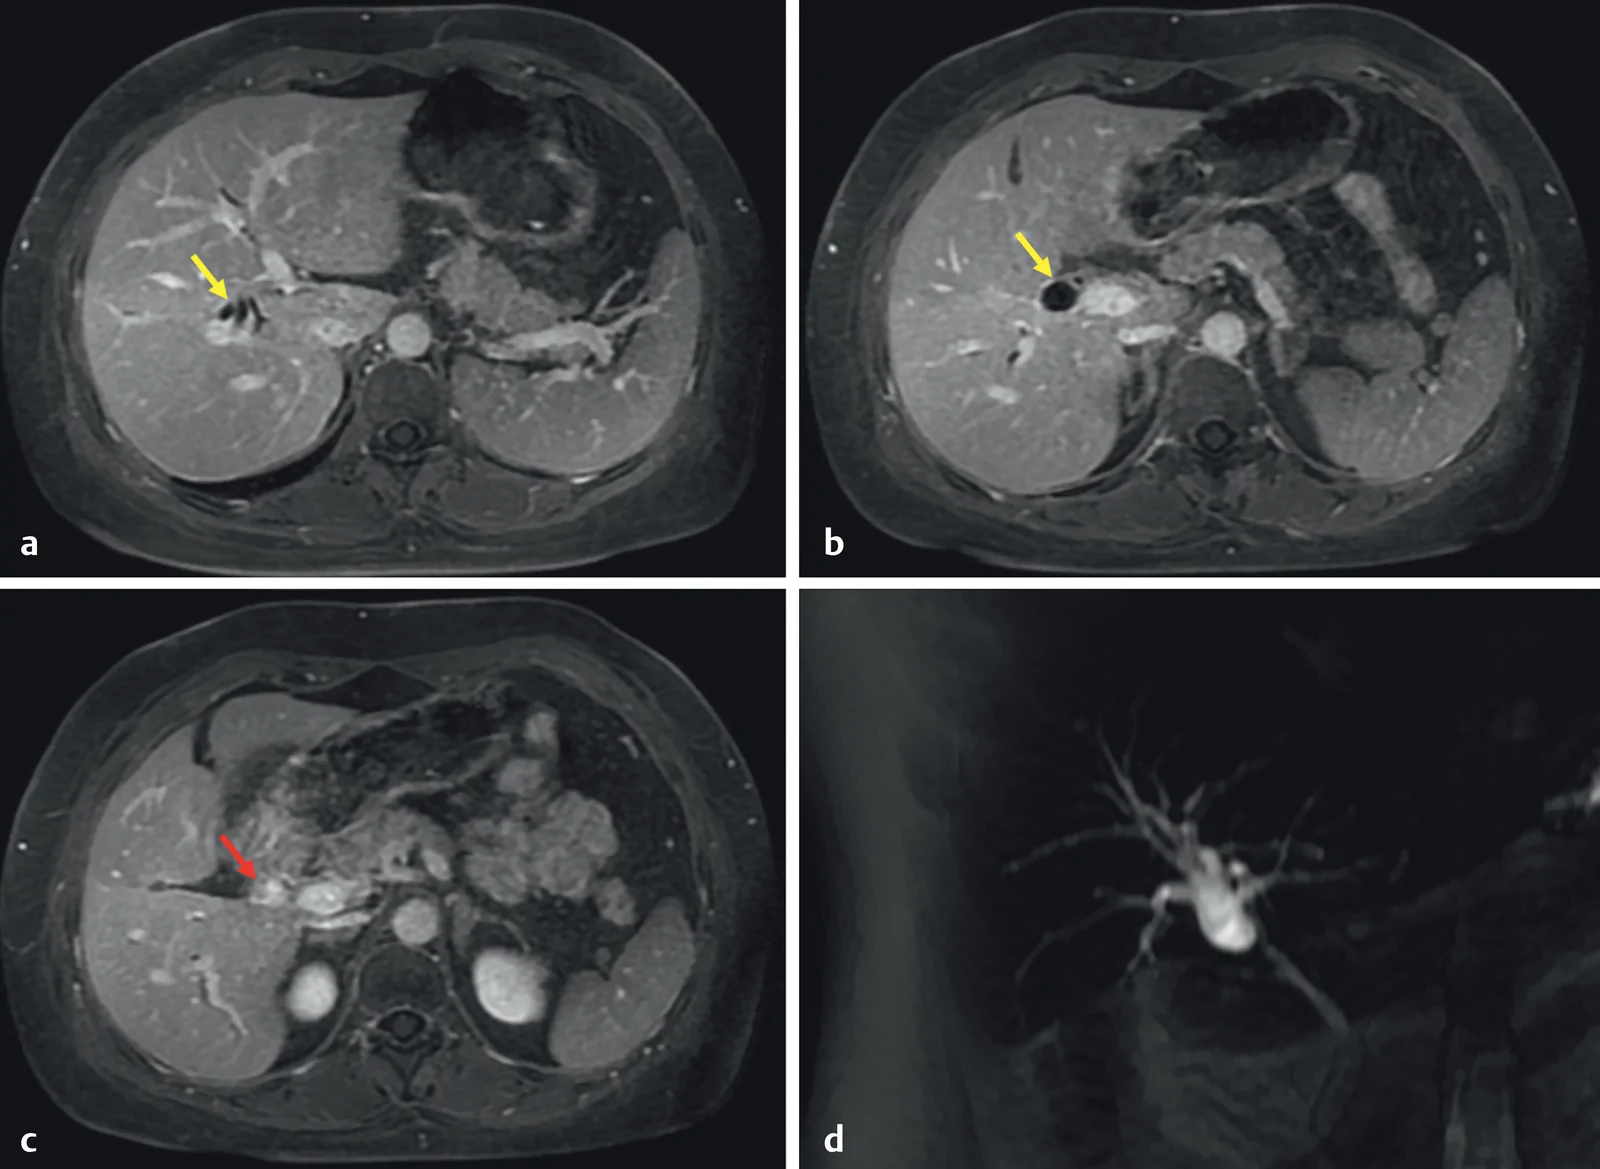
Preoperative MRI findings. (
